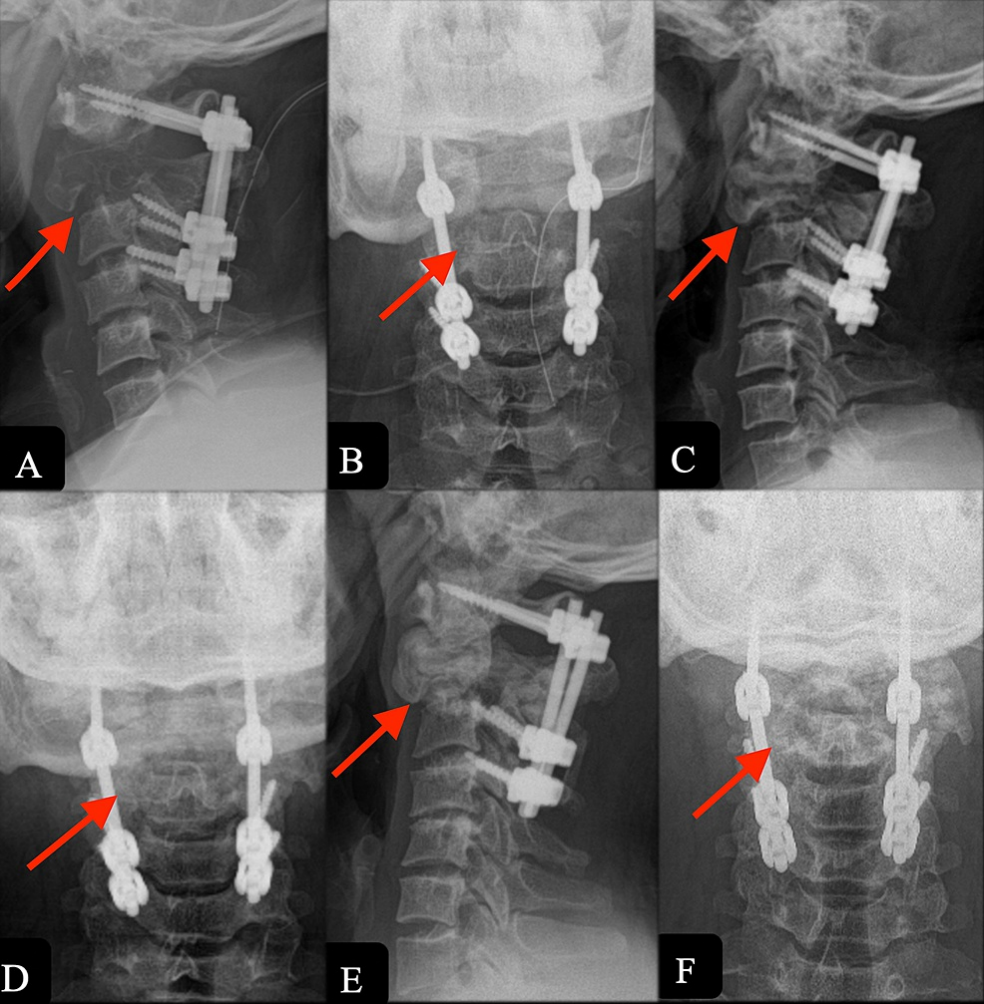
Immediate postoperative lateral and anteroposterior radiographs (A, B) showed tumor resection and posterior fixation from C1-C4. One-year follow-up radiographs (C, D) showed restoration of C2 vertebra with partial sclerosis. Two-year follow-up radiographs (E, F) show complete sclerosis with no evidence of lysis.
